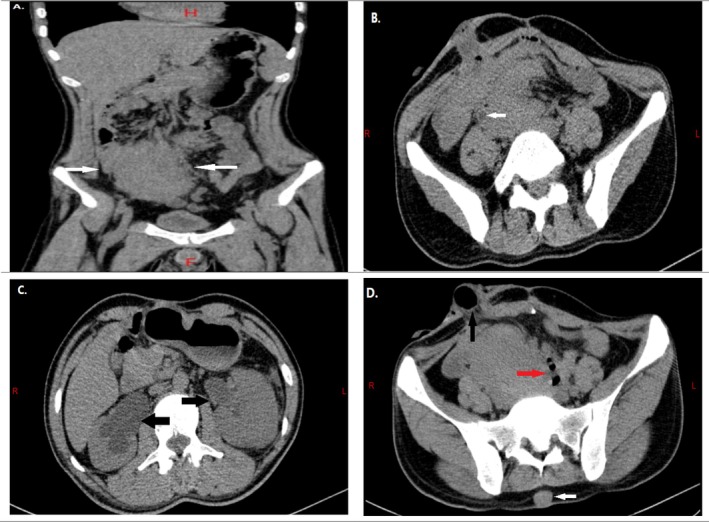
(A) Non‐contrast coronal abdominopelvic CT image demonstrating a large, infiltrative mesenteric mass (between white arrows) in the lower abdomen and pelvis, consistent with a desmoid tumor. The mass infiltrates the greater omentum, displaces small bowel loops, invades the right ureter, and compresses the left ureter, leading to moderate bilateral hydroureteronephrosis. (B) Non‐contrast axial abdominopelvic CT image, the mass is seen encasing and invading the right ureter (white arrow). (C) Non‐contrast axial abdominopelvic CT image demonstrating bilateral hydroureteronephrosis (black arrows), with visible dilation of both renal pelvicalyceal systems and proximal ureters. The findings are consistent with obstructive uropathy, attributed to an infiltrative mesenteric desmoid tumor encasing and compressing the ureters. (D) Non‐contrast axial abdominopelvic CT image showing post‐surgical changes consistent with prior total colectomy, including absence of colonic structures and evidence of stoma placement (black arrow) in the right abdominal wall. A large, ill‐defined desmoid tumor measuring approximately 11 × 7 × 12 cm is seen occupying the lower abdomen and pelvic cavity, with infiltration and displacement of adjacent small bowel loops (red arrow) and involvement of the right ureter, contributing to obstructive pyelonephritis. Additionally, a well‐defined, subcutaneous, 1 × 1 × 1 cm soft tissue lesion is noted in the posterior midline over the sacral region (white arrow), suggestive of a subcutaneous epidermoid cyst.
